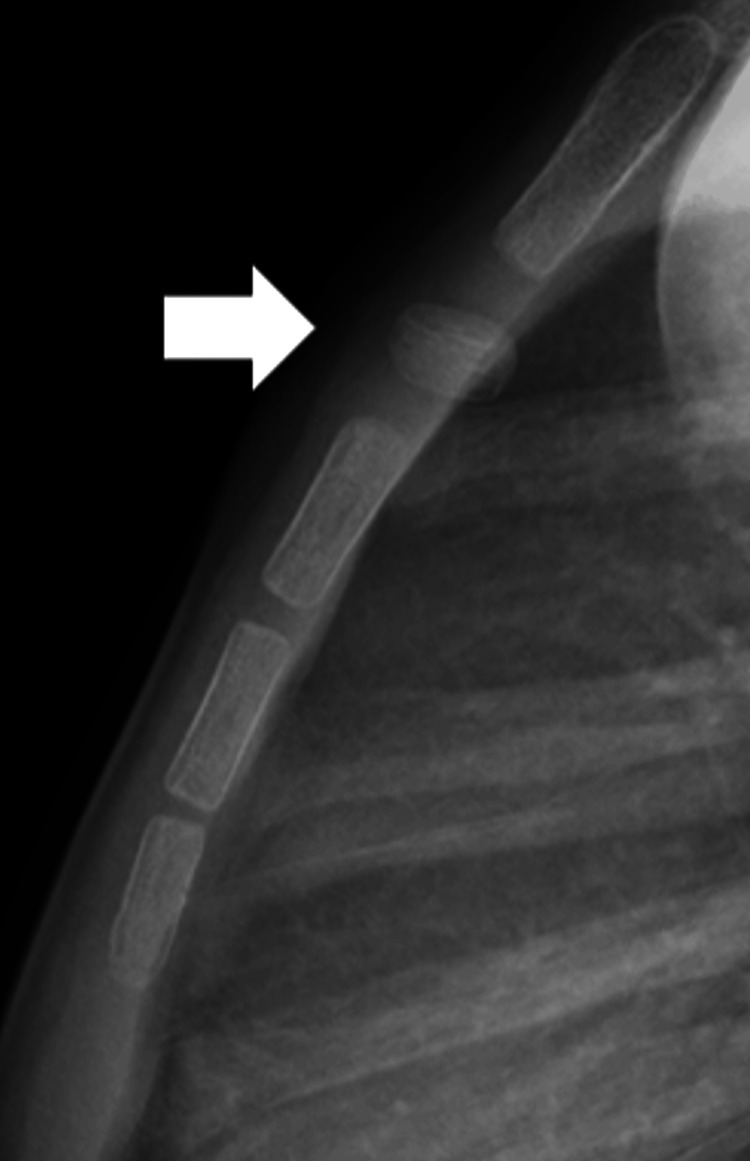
Sternal segment dislocation without anterior displacement on radiograph (white arrow).
